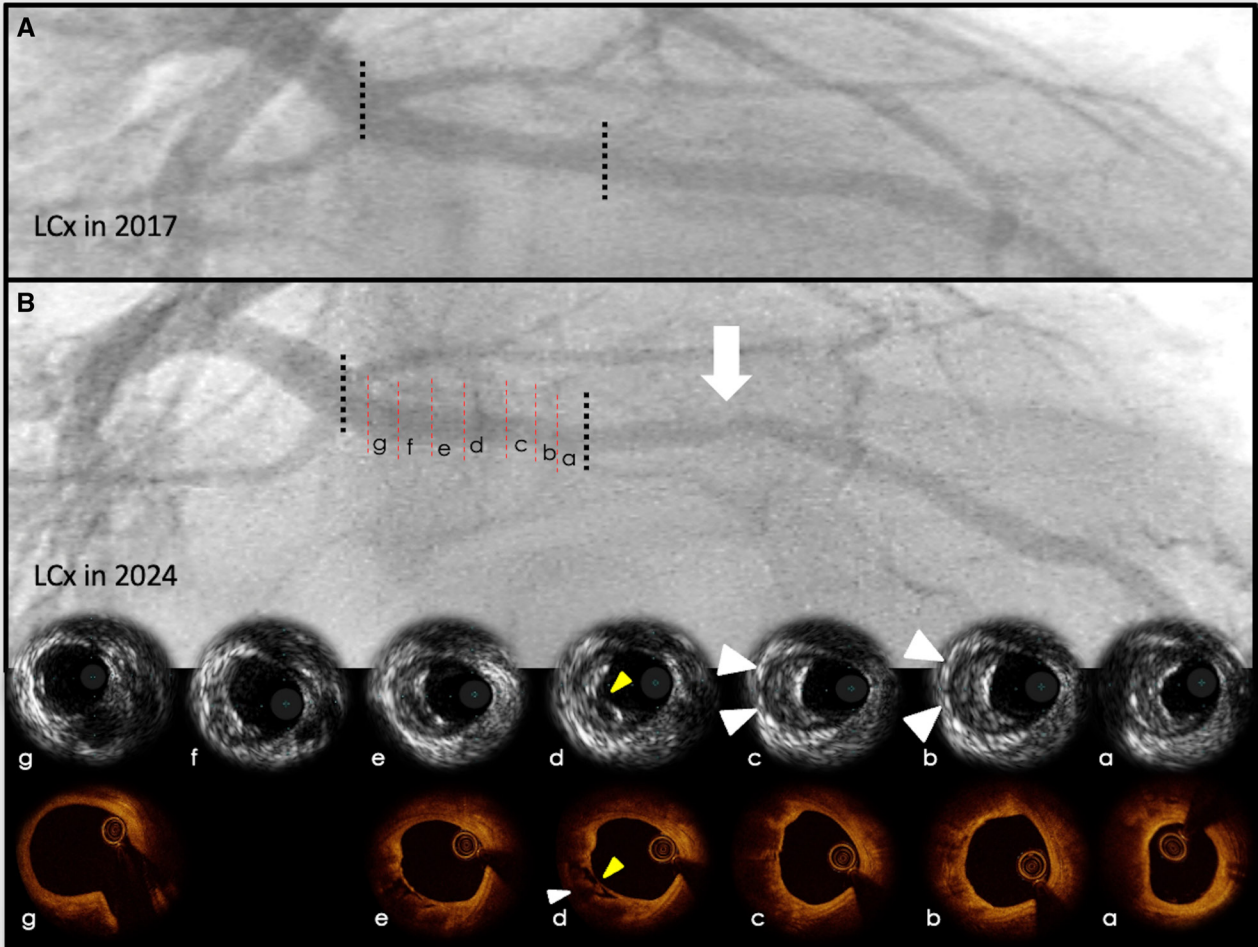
Male patient with multiple cardiovascular risk factors at baseline and follow-up. A, A 41-year-old male patient with multiple cardiovascular risk factors and mild atherosclerosis of the left circumflex (LCx). B, Seven years later, the patient presented with unstable angina. Percutaneous coronary intervention revealed severe stenosis of the mid portion of LCx (white arrow). Intravascular ultrasound images of the segment proximal to the stenosis are shown above; note the presence of a nonobstructive atherosclerotic plaque with positive remodeling (white arrowheads) and ulceration (yellow arrowhead). Optical coherence tomography, performed at the same level, is shown below; note the presence of thin-cap fibroatheroma (yellow arrowhead) and necrotic core (white arrowhead).
